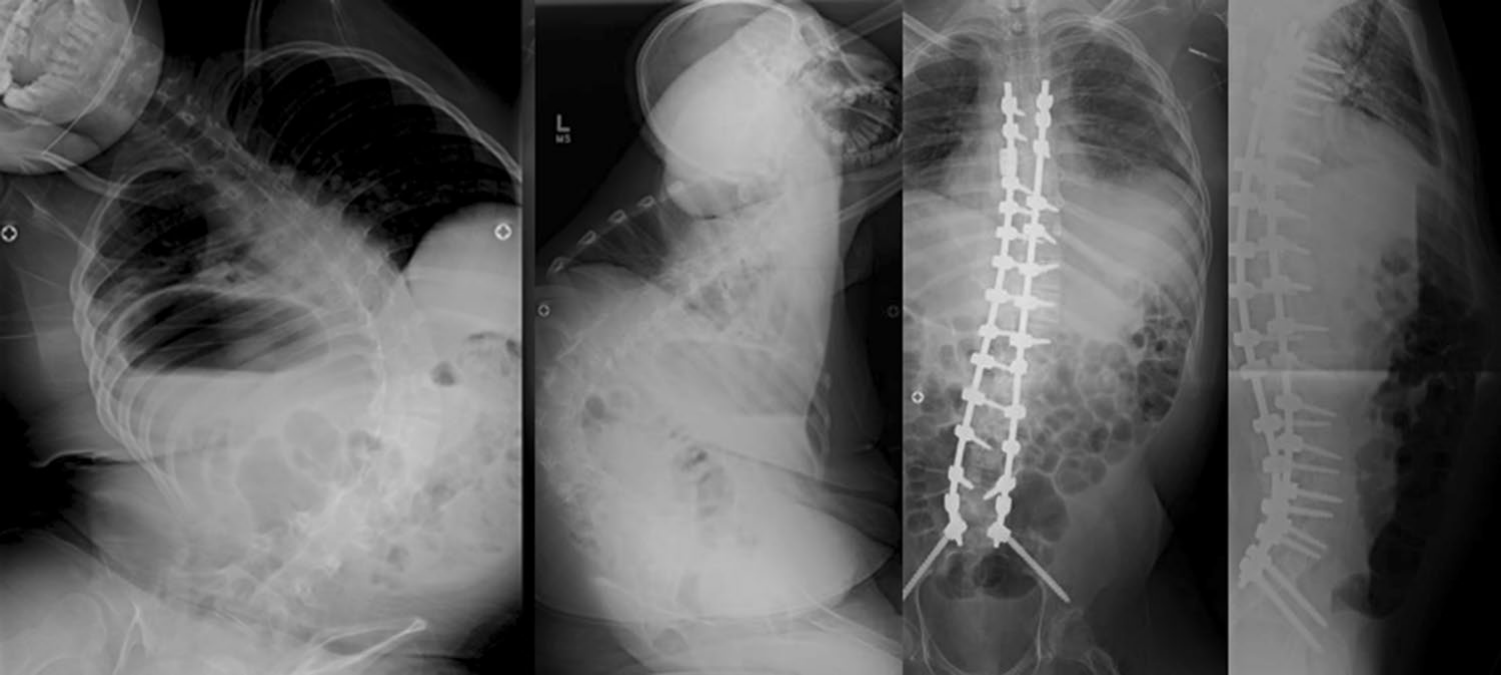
Fifteen-year-old male with a coronal curve magnitude 111-degree neuromuscular scoliosis secondary to Duchenne’s muscular dystrophy and C-DAR = 11, S-DAR, 10, T-DAR = 21. This patient was treated with PSF from T5-pelvis without vertebral column resection or three-column osteotomy. IONM signals remained stable throughout surgery and there were no instances of IONM signal loss. Follow-up radiographs at 1-month postop reveal coronal correction of the spinal deformity
